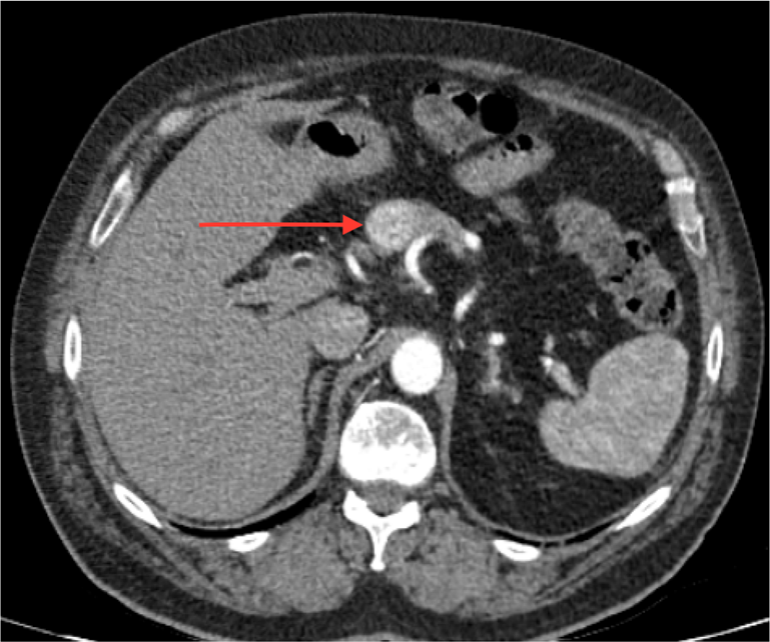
Hypervascular pancreatic metastasis from renal cell carcinoma (*arrow*), not reported on CT; lung and mediastinal nodal metastases identified and reported (satisfaction of search error)
